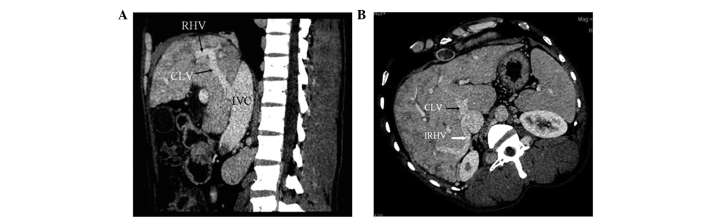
HV-accessory HV collaterals. (A) Computed tomography angiography shows segmental occlusion of the RHV. Blood drains into the IVC through the dilated CLV. (B) Blood drains into the IVC through the dilated IRHV and CLV simultaneously in another patient. HV, heptatic vein; RHV, right HV; IVC, inferior vena cava; CLV, caudate lobe vein; IRHV, inferior right HV.
